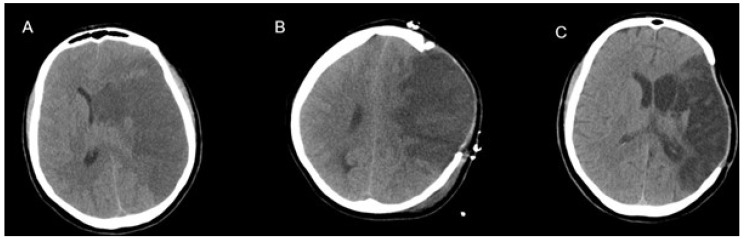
Computed tomography (CT) scans of a 37-year-old female patient with left-sided MMCAI (malignant middle cerebral artery infarction) (A). Postoperative 1st day CT (B). Postoperative third week CT showed no midline shift (C).
